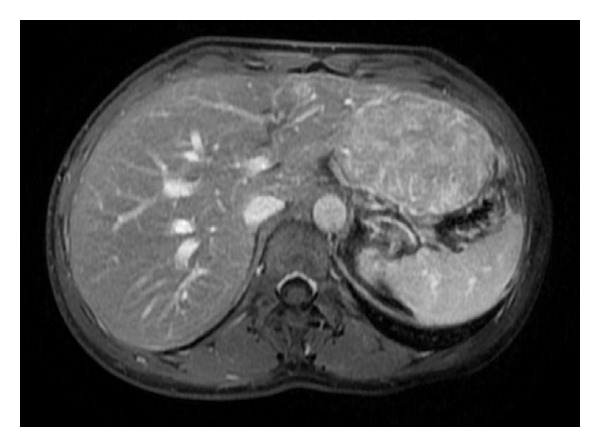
Focal nodular hyperplasia in gadolinium-enhanced MRI (contrast medium: gadoxetic acid; disodium salt (Primovist, Eurokontrast GmbH, Heidelberg), scanner: GE Signa HDxt 1,5T (General Electric Company, USA)): inhomogeneous hyperintensity on T1 FS in portal-venous contrast phase (T1 FSPGR FS FA12, TR 4,24 TE 2,04, TI 7).
